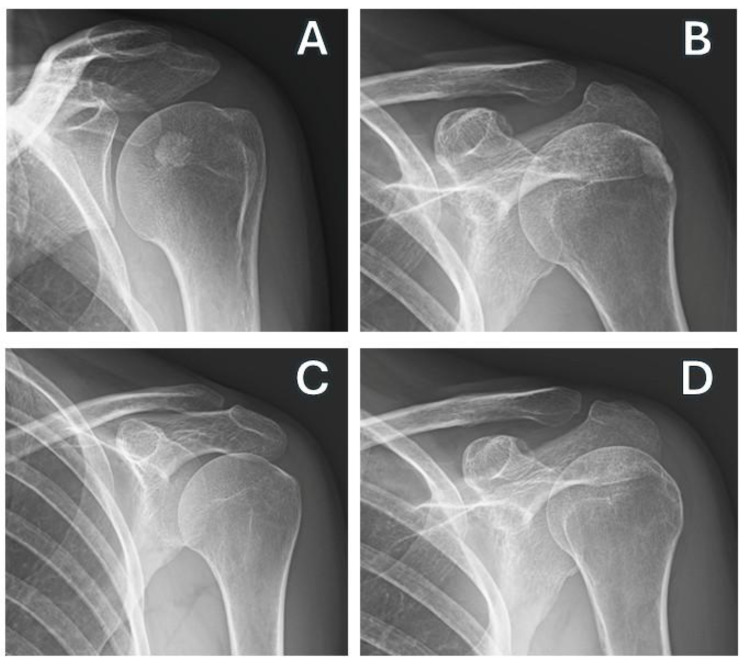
(A,B) Conventional x-rays, pre-treatment images. (C,D) Conventional x-rays, post-treatment images.
